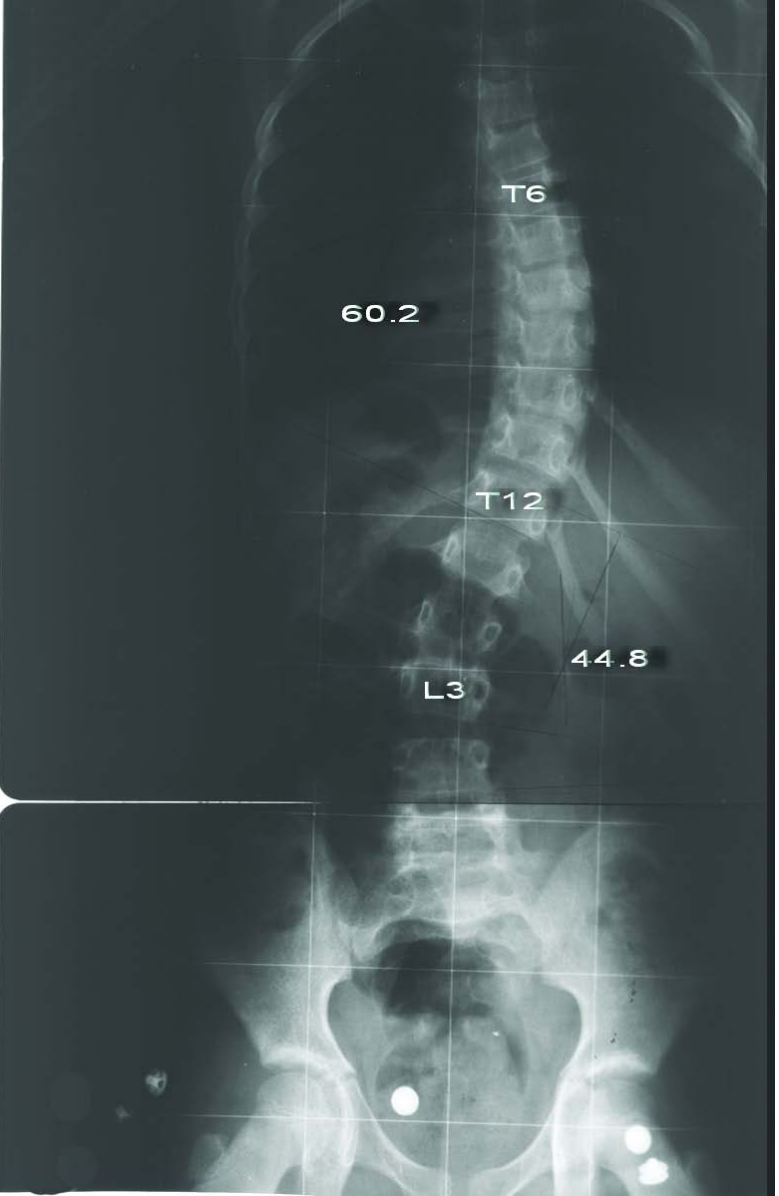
Eight-year-old girl with Lenke type 3BN scoliosis (Patient no 1). Preoperative anteroposterior radiograph shows 60.2° main thoracic curve and 44.8° lumbar curve.
